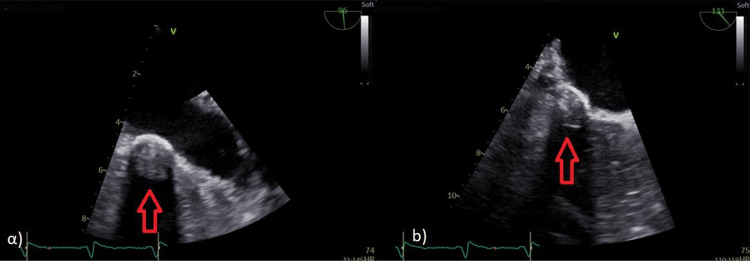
Transesophageal echocardiography. Red arrows show the echogenic mass on the posterior mitral annulus.
